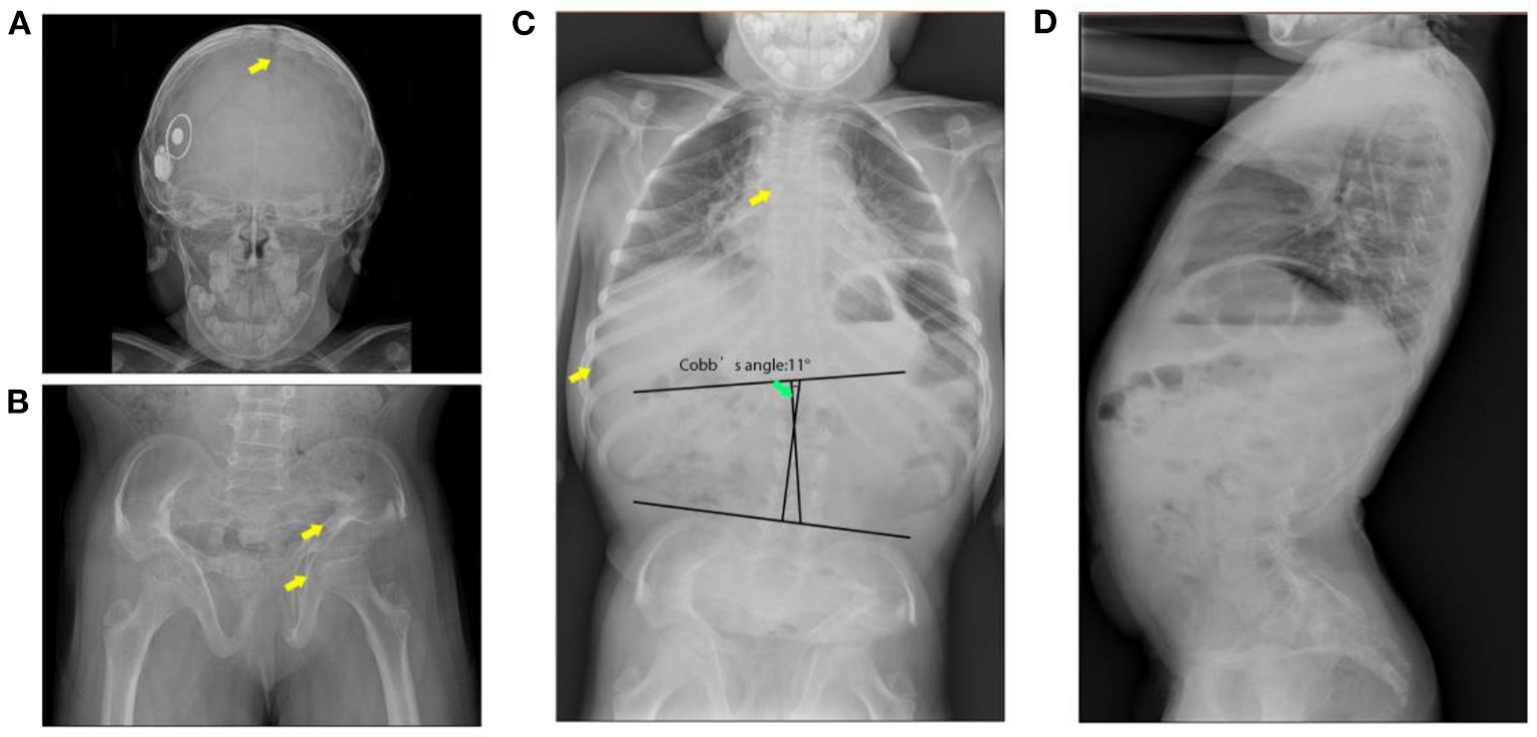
X-ray images of the patient. (A) Broad skull with open suture and wormain bones (indicates with the arrow). (B) Malformation of the pelvis (indicates with the arrow), low bone density, wide and flat acetabulum (indicates with the arrow), and increased angle of the femoral neck stem. (C) Flattened spinal vertebrae (indicates with arrow), thin ribs, and scoliosis. (D) Pronounced anterior lumbar convexity.
